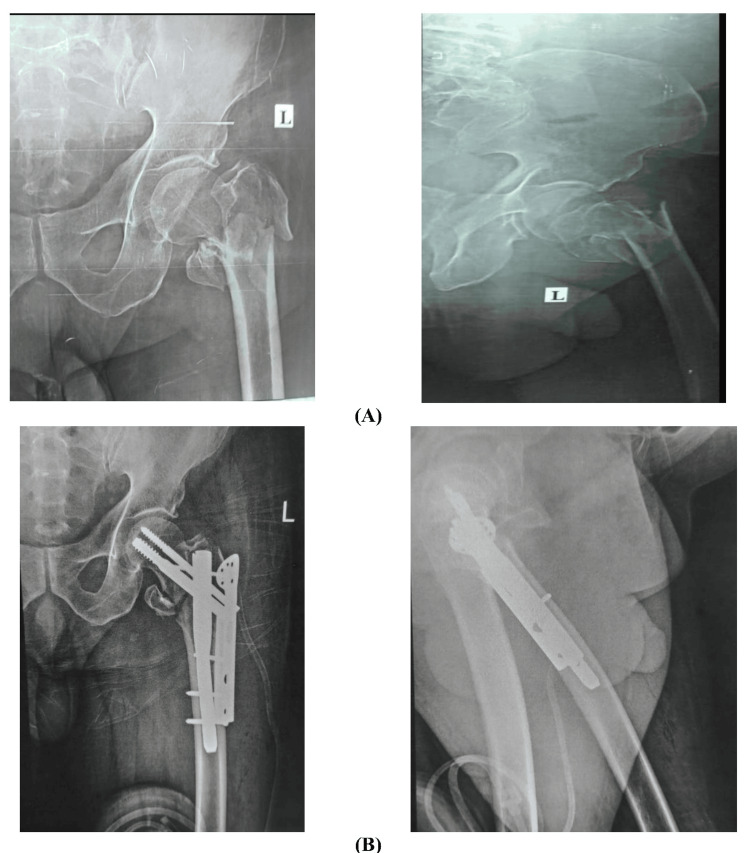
Short nail usage (A) Preoperative X-rays show an unstable inter-trochanteric femur fracture. (B) Postoperative anteroposterior and lateral X-ray shows a nicely reduced fracture.
